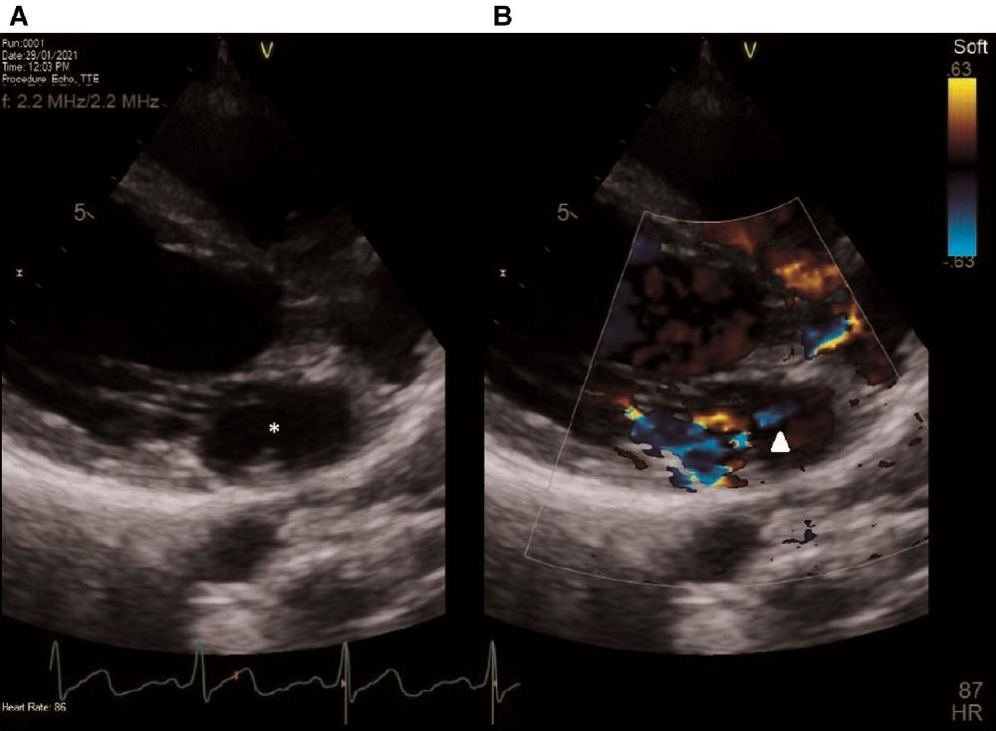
Subsequent transthoracic echocardiography. Two-dimensional parasternal modified long-axis, simultaneous colour Doppler view. (*A*) The left atrium was depicted (asterisk). (*B*) There appeared to be a jet directed inside the left atrium (arrowhead), raising the suspicion of an unroofed coronary sinus.
